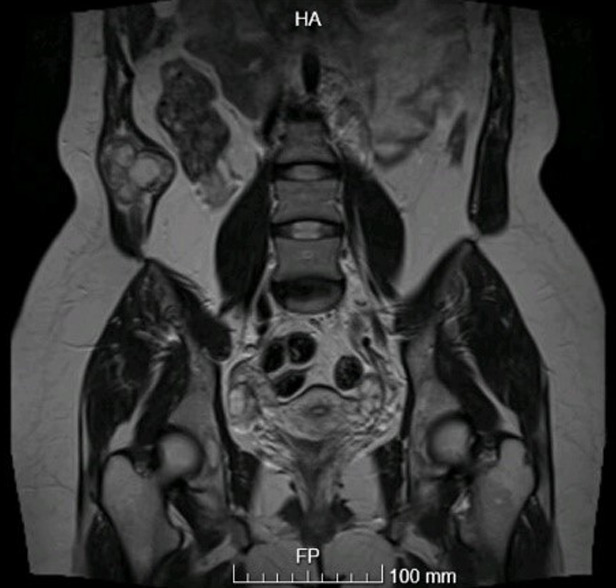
MRI abdomen showing the abdominal wall mass; a multiloculated cystic mass along the right lower anterior abdominal wall muscles (with cysts within cysts) measuring 9 x 8.5 x 4.7 cm
